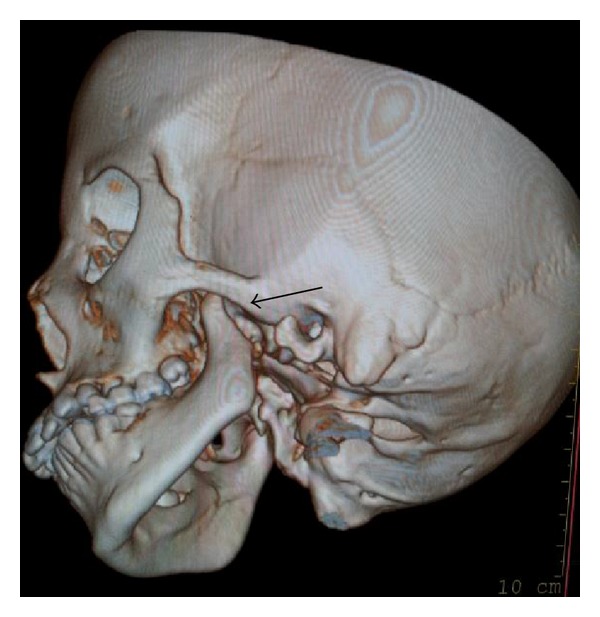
3D CT scan of affected side (left) with missing condyle and glenoid fossa.
